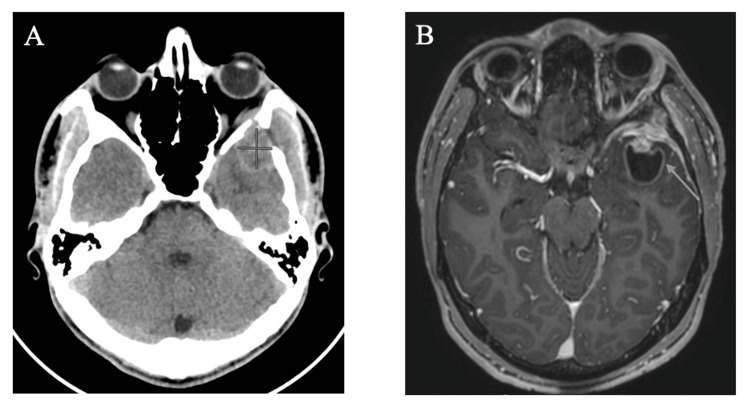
Initial CT head and MRI brain findings. (A) CT head shows an intracranial mass centered in the left greater sphenoid area; (B) MRI brain shows the same mass measuring 2.9 cm x 2.3 cm x 2.6 cm, extending into the surrounding area. CT, computed tomography; MRI, magnetic resonance imaging
